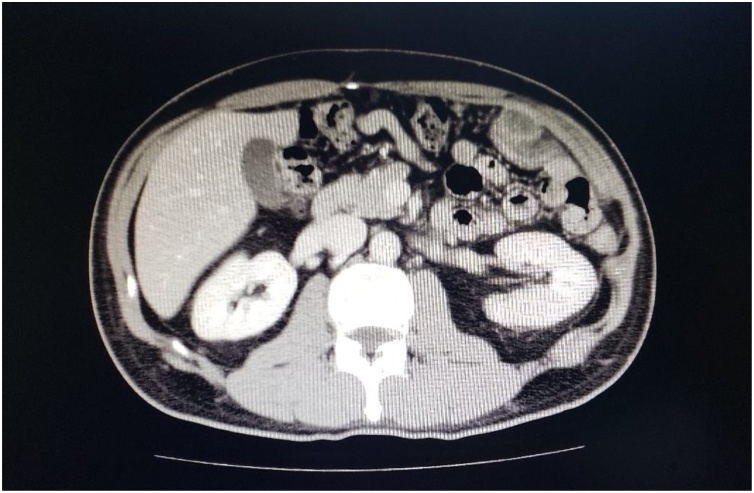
CT scan of the last follow up show no recurrent disease.
